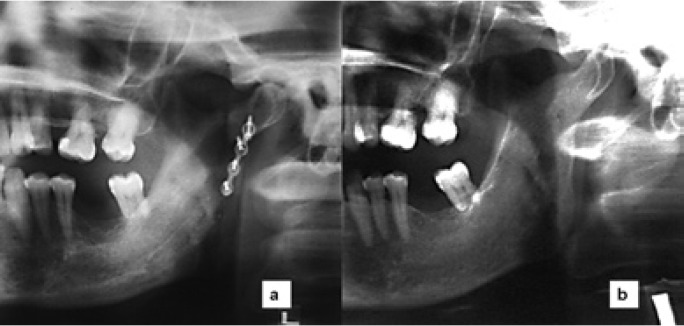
a) Postoperative panoramic radiograph showing one miniplate of osteosynthesis. b) Panoramic radiograph after 5 years of follow up.
